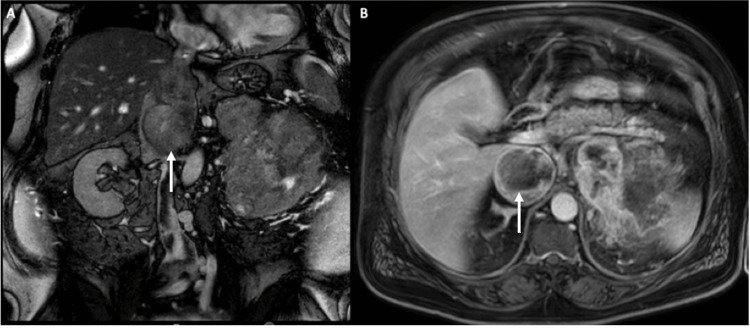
MRI showing coronal (A) and axial (B) views of the left renal mass with thrombus (white arrows) invading the left renal vein and up to hepatic confluence in the IVC MRI: magnetic resonance imaging; IVC: inferior vena cava
